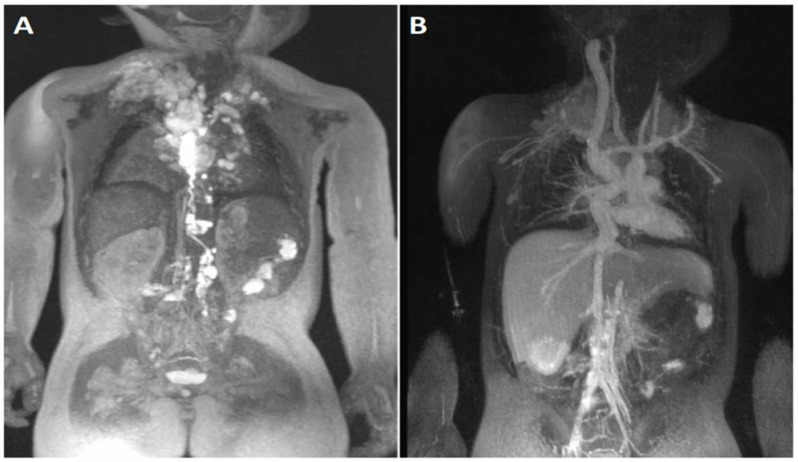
Combined MR Lymphangiography and FE-MRA. Panel (A): Coronal maximum intensity projection of a breath-held T2-weighted MR lymphangiogram performed after administration of dilute gadolinium via groin cannula. Panel (B): Coronal maximum intensity projection of a breath-held FE-MRA is also obtained to obtain the vascular anatomy and “overlay” the two images to guide transcatheter lymphatic therapy.
